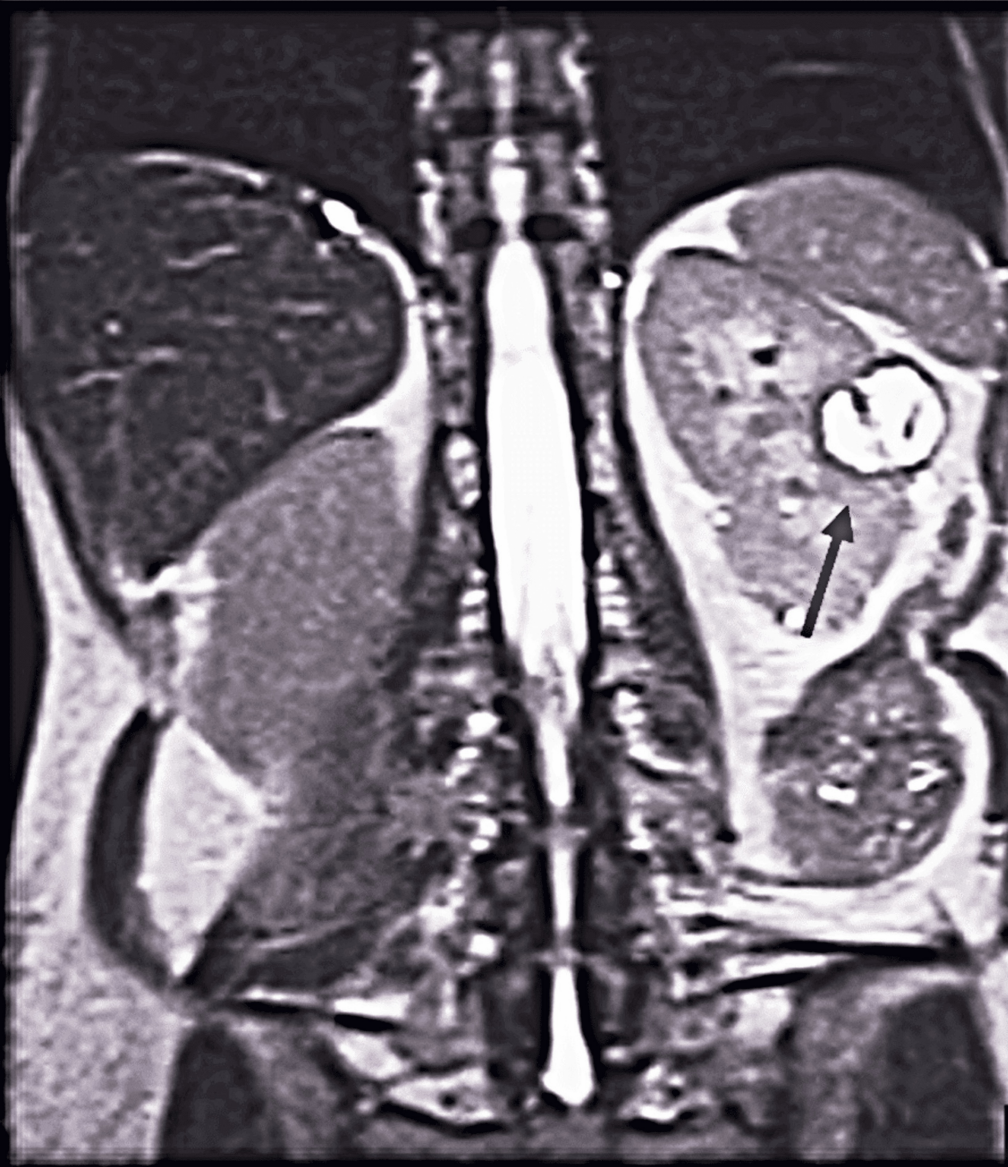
Renal MRI scan showing a 3 cm left renal mass
